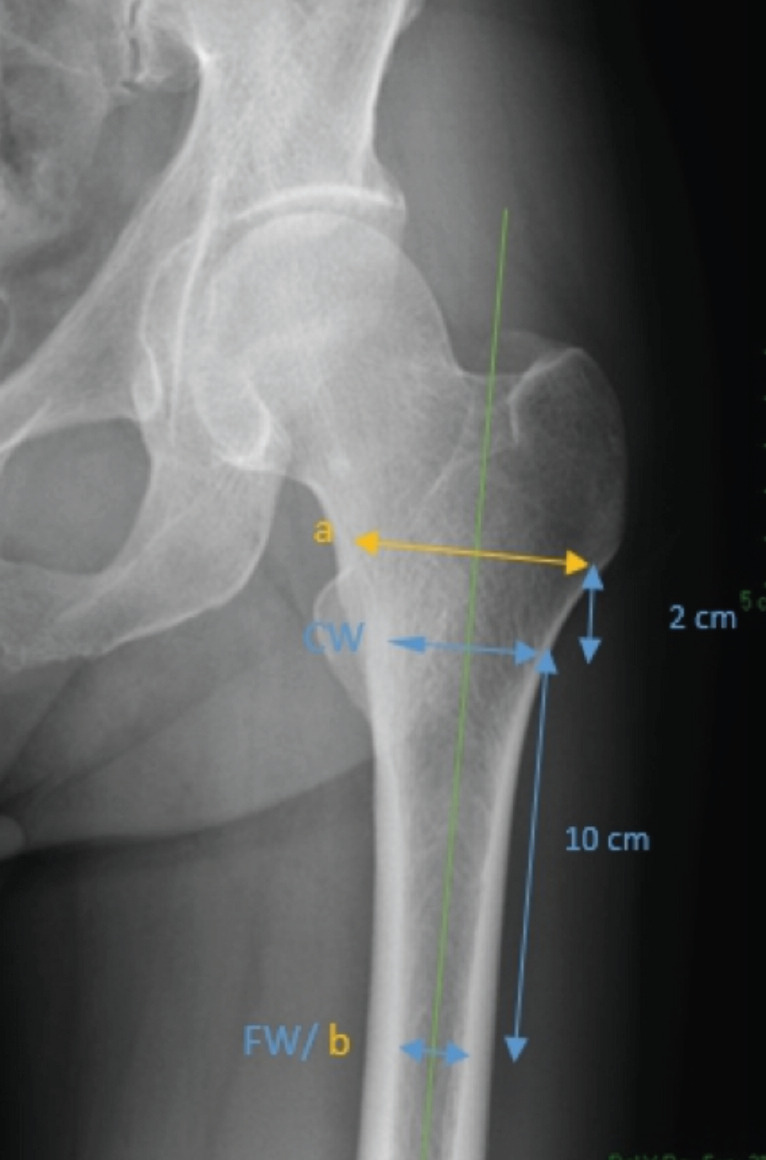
Anteroposterior pre-operative radiograph of a right hip showing determination CFI and Dorr score.
